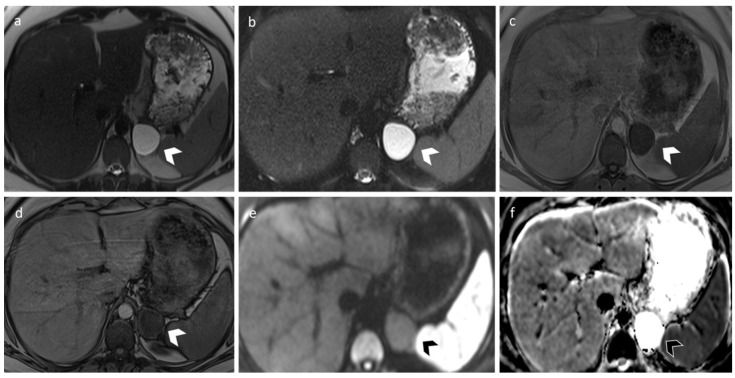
A 33-year-old female with an incidental left adrenal cyst. MR revealed a 4 cm nodular lesion in the left adrenal gland characterized by thin walls and high and homogeneous signal in T2 (a) and T2 fat-sat sequences (b). T1 signal (c,d) is in accordance with simple fluid content, and DWI/ADC sequence (e,f) does not show signs of hypercellularity.
